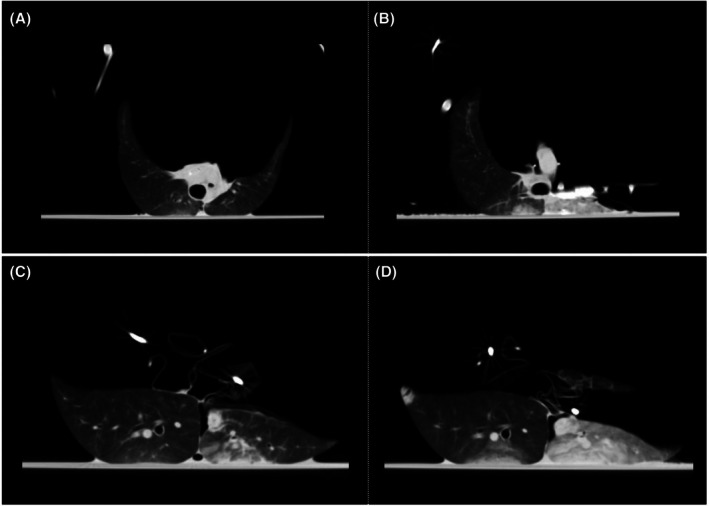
Comparison between the same portion of the lung at the computer tomography (CT) scan analysis at baseline (A) and during left superior bronchial occlusion (B), and at baseline (C) compared to left inferior bronchial occlusion (D).
